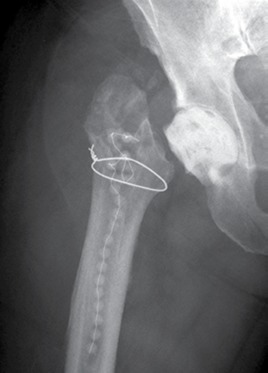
Postoperative x-ray reveals the antibiotic beads and the cement spacer after removal of the infected implant.
